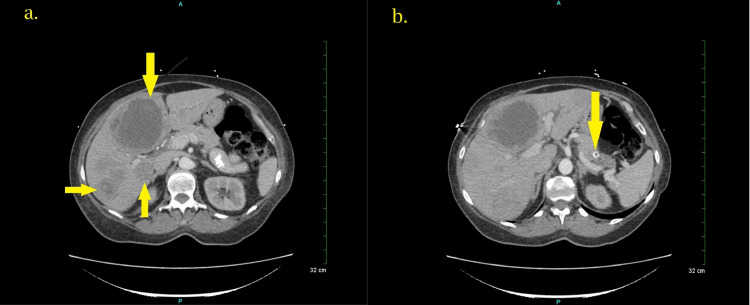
CT scan images of the abdomen with contrast: Multiple hepatic lesions measuring up to 8.2 cm are seen in the image on the left (a), and a 9 mm nodule is identified in the pancreatic tail with a rim seen in the image on the right (b).
